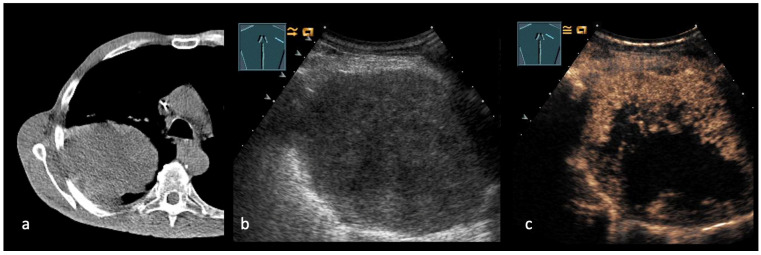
(a–c): CT-scan of a lung cancer (a). B-US presents a round, hypoechoic pulmonal lesion (b) with a large central, necrotic area in CEUS (c).
